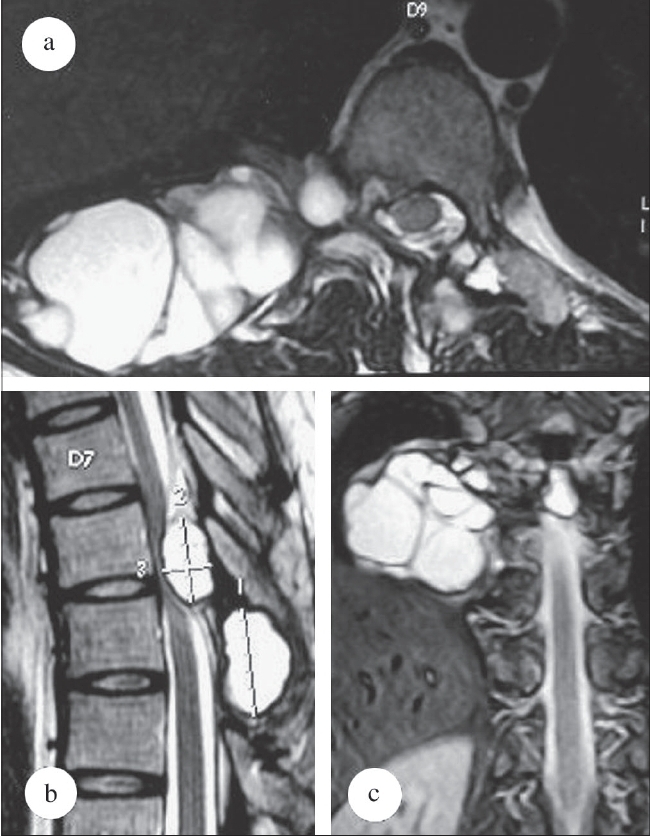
Preoperative MRI images T2 Axial (a) Sagittal (b) and coronal (c) images showing intraspinal and extraspinal cysts
